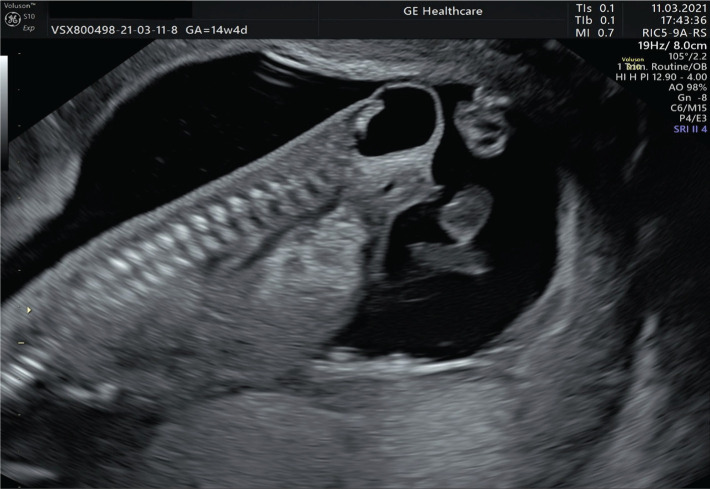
Meningocele at 14 weeks of gestation (2D ultrasonography).
